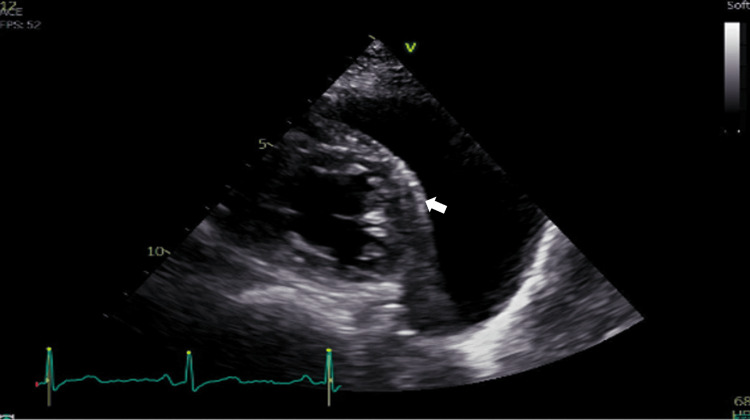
Transthoracic echocardiogram showing a moderate-sized liquid collection located along the left side wall of the heart (arrow) with possible diagnosis between pericardial effusion or pericardial cyst (echocardiogram limited for extracardiac lesion characterization). This examination confirmed normal biventricular dimensions and function, with no hemodynamic compression by the collection.
